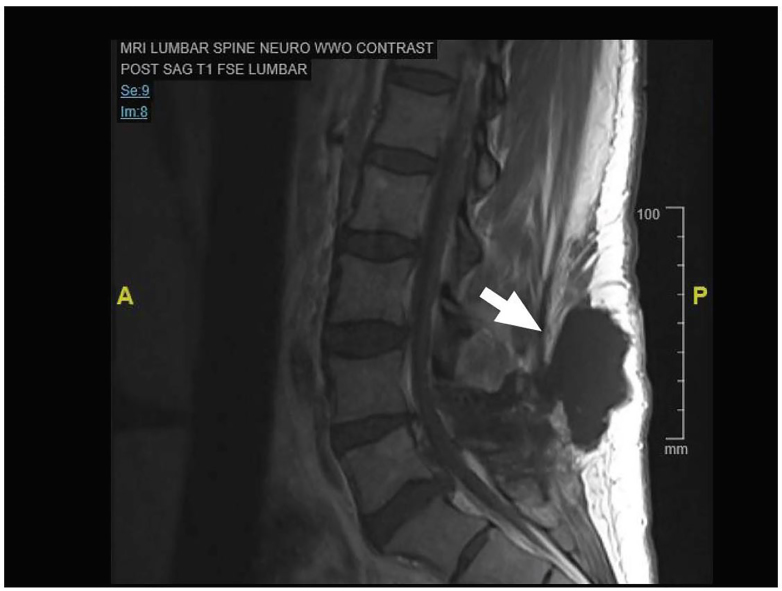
Sagittal T1-weighted magnetic resonance imaging of the lumbosacral spine demonstrating the pseudomeningocele (arrow) identified initially on ultrasound.
